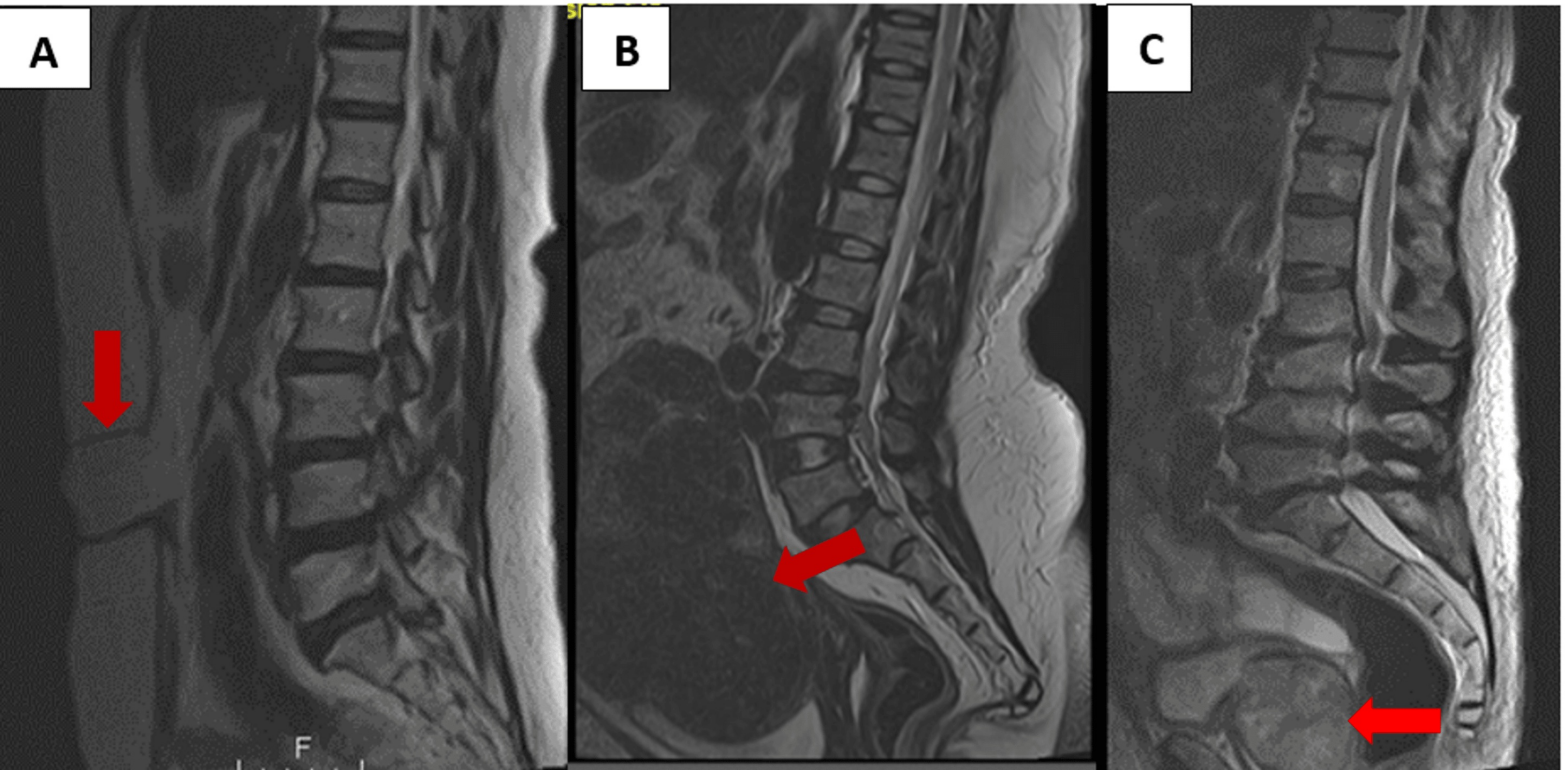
(A) Unenhanced sagittal T2W MR image of the lumbosacral spine of a 52-year-old man showing an anterior abdominal wall defect. (B) Unenhanced sagittal T2W MR image of the lumbosacral spine of a 40-year-old woman showing an enlarged anteverted uterus with multiple uterine fibroids of varying sizes and (C) unenhanced sagittal T2W MR image of the lumbosacral spine of a 65-year-old man showing an enlarged heterogeneous prostate gland. All depicted with the red arrows.
